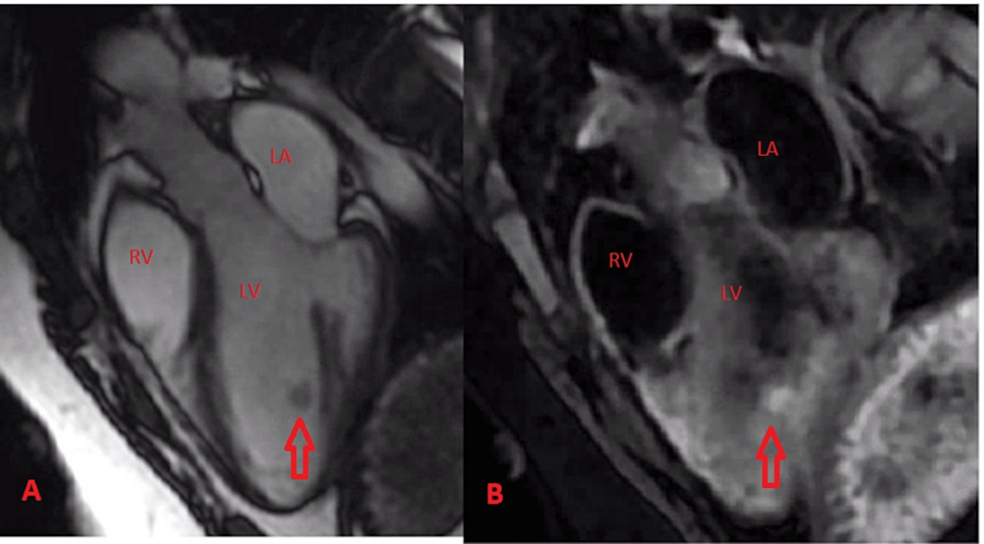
Cardiac MRI imaging A. Steady-state free precession (SSFP) imaging in a 3-chamber orientation demonstrates the mass to be isointense to the myocardium. B. Fat-suppressed black blood T2 weighted imaging (DIR spin echo) demonstrating the mass to by hyperintense compared to the myocardium. LV: Left ventricle, LA: Left atrium, RV: Right Ventricle, DIR: Double inversion recovery
